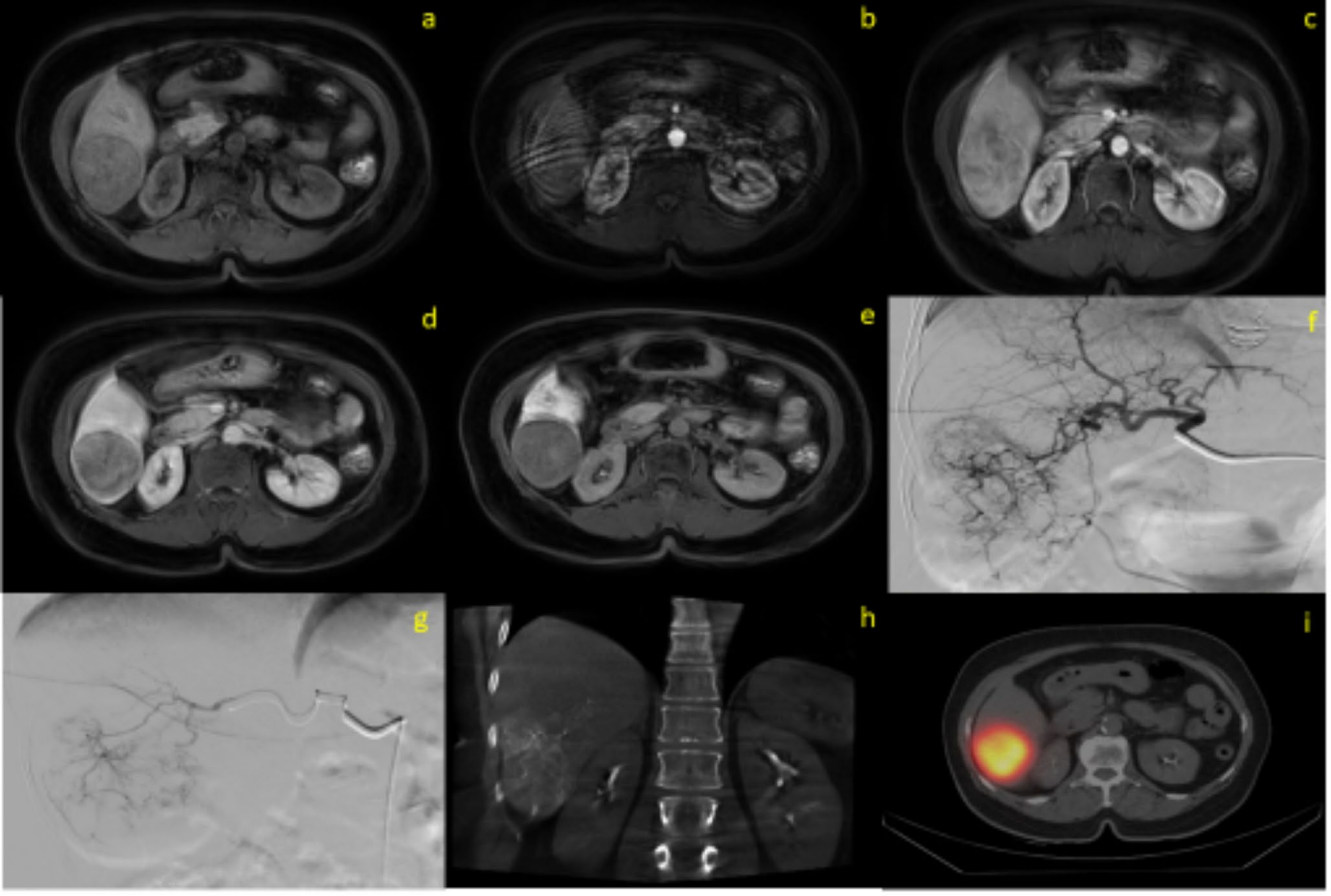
Imaging of a 63-year-old female patient with an 46 mm HCC lesion, demonstrating MAA uptake in segment 6. (a-e) Precontrast phase (a), Arterial phase (b), Portal venous phase (c), Venous phase (d) and Hepatobiliary phase (e) contrast-enhanced T1-weighted MRI image of the lesion in segment 6; (f, g) Angiographic images of the main hepatic artery (f) and the segmental artery with MAA administration (g); (h) Cone beam CT image of the segmental artery where MAA was administered; (i) SPECT imaging after MAA injection. (CT: Computed tomography; HCC: Hepatocellular carcinoma; MAA: Tc-99 m-macroaggregated albumin; MRI: Magnetic resonance imaging; SPECT: Single-photon emission computed tomography)
